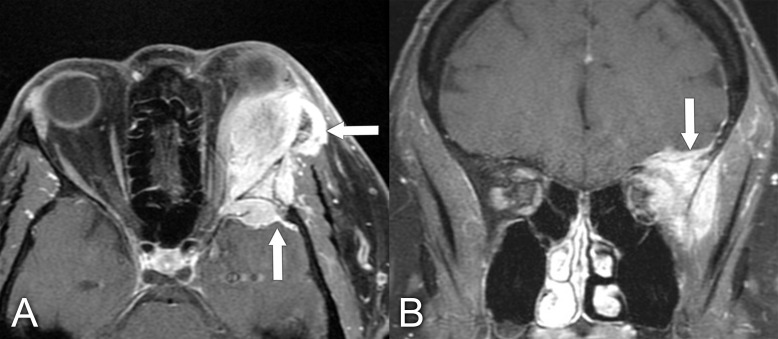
MRI findings in a 59-year-old man with locally advanced (T4) adenoid cystic carcinoma of the lacrimal gland. (A) Axial T1 postcontrast MRI with fat saturation shows lateral extension of the mass into the temporal fossa and posterior extension into the middle cranial fossa (arrows). (B) Coronal T1 postcontrast MRI with fat saturation shows extension of the lesion into the anterior cranial fossa (arrow).
